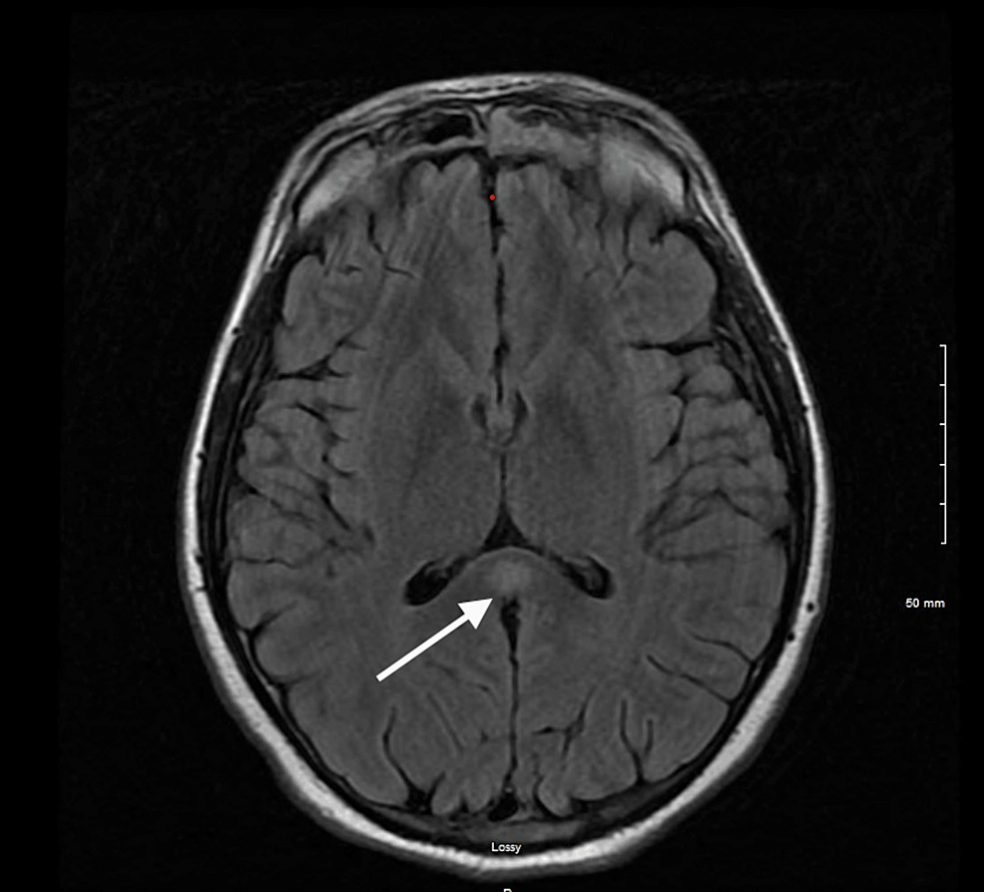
Axial T2-FLAIR MR image showing a high signal intensity area in the central part of the corpus callosum FLAIR: fluid-attenuated inversion recovery
